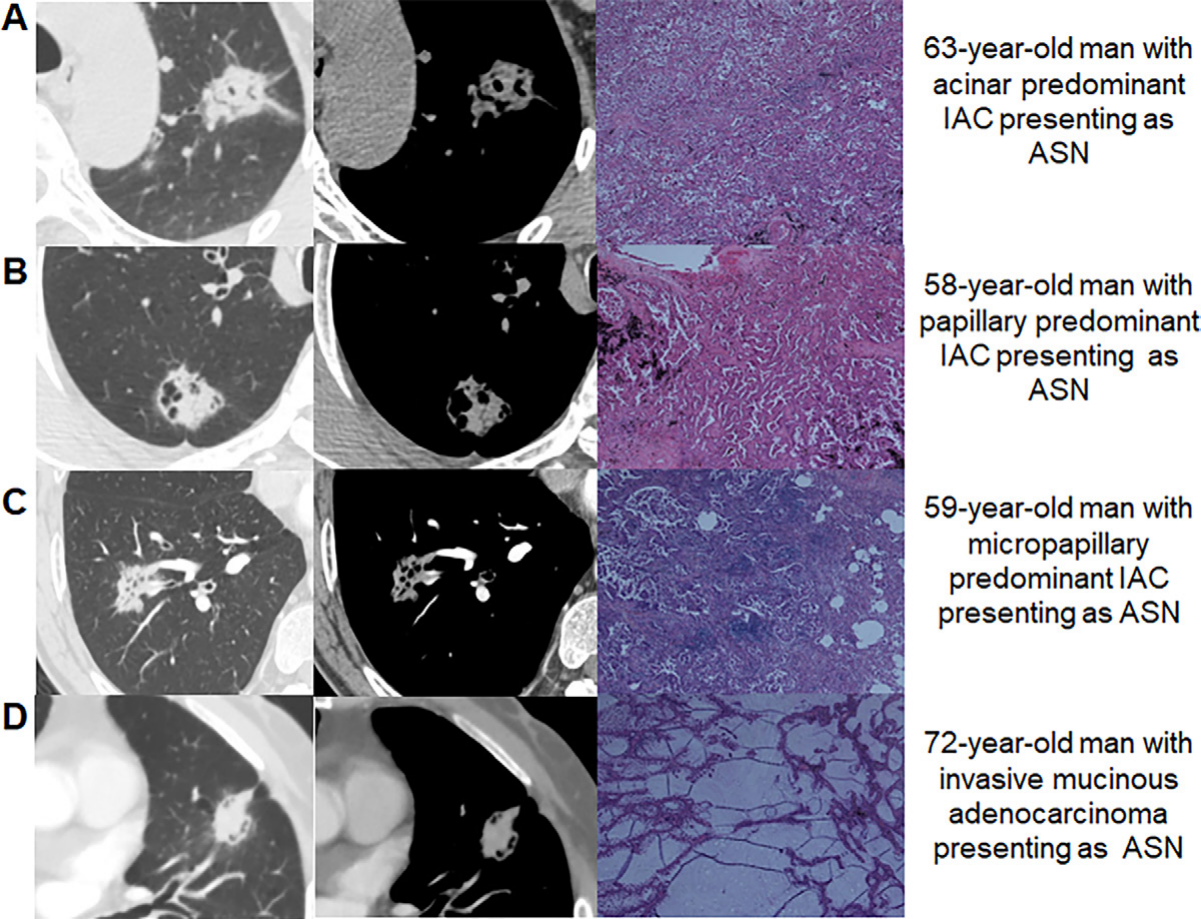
CT and pathologic findings of atypical solid nodules (ASNs).
